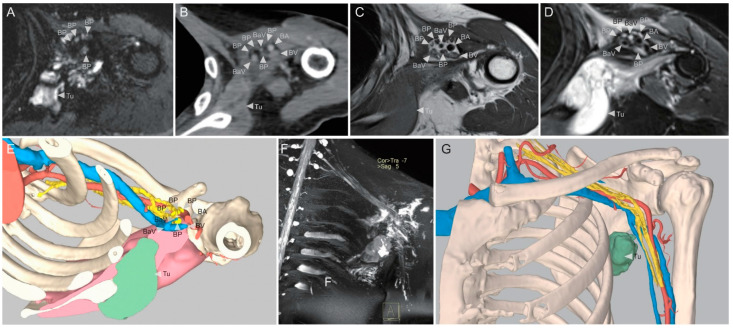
Classic images and their 3D reconstructions in a patient with liposarcoma. (A) MR hydrography image; (B) CT angiography image; (C) T1-weighted MRI; (D) T2-weighted MRI; (E) multimodality 3D reconstruction image with similar axial views as (A–D); (F) coronary view of MR hydrography image; (G) anterior view image of multimodality 3D reconstruction. Abbreviations: BA, brachial artery; BaV, basilic vein; BV, brachial vein; BP, brachial plexus; Tu, tumour.
